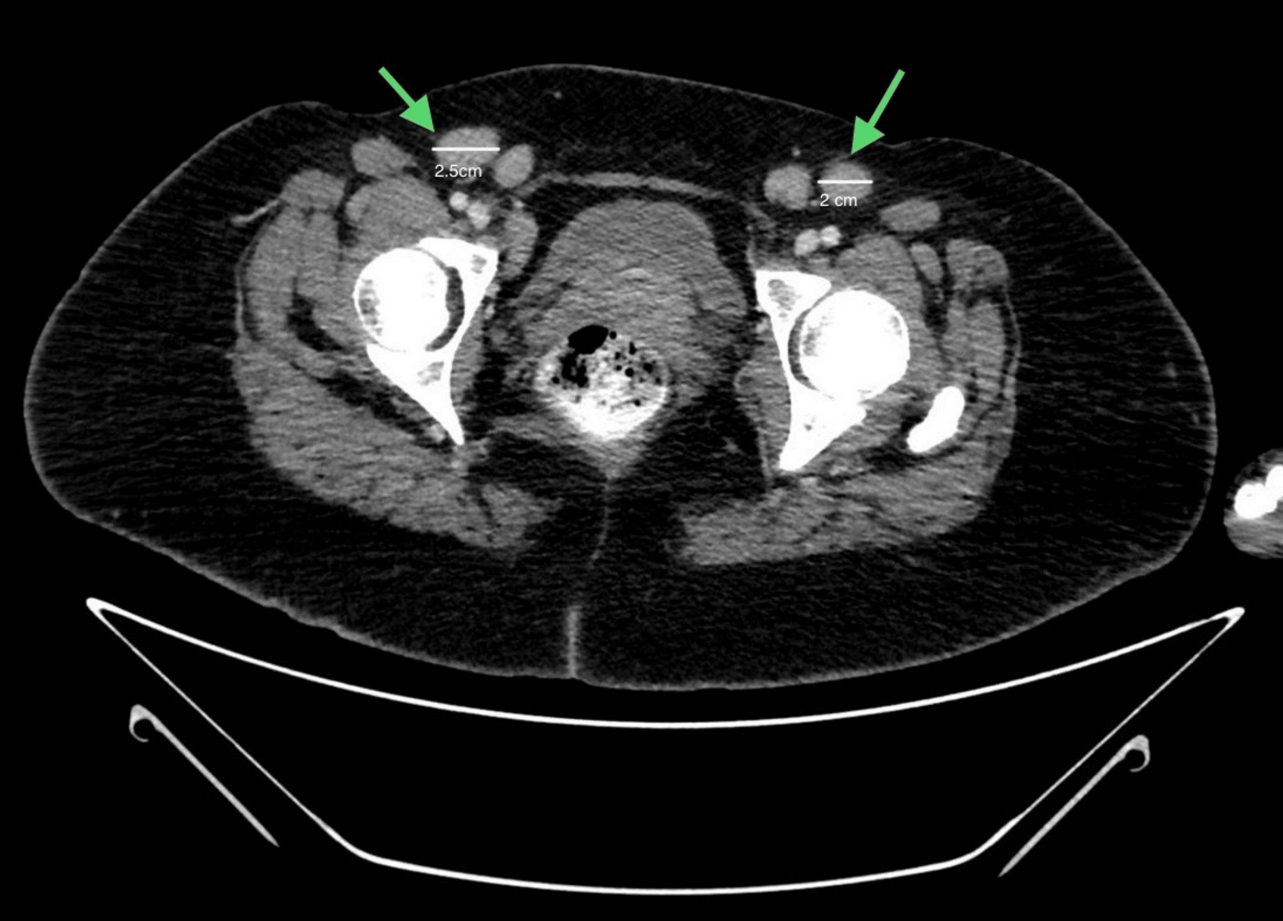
Pan-CT revealing multiple bilateral inguinal enhancing lymph nodes (scale bar: 2.5 cm) CT: computed tomography
